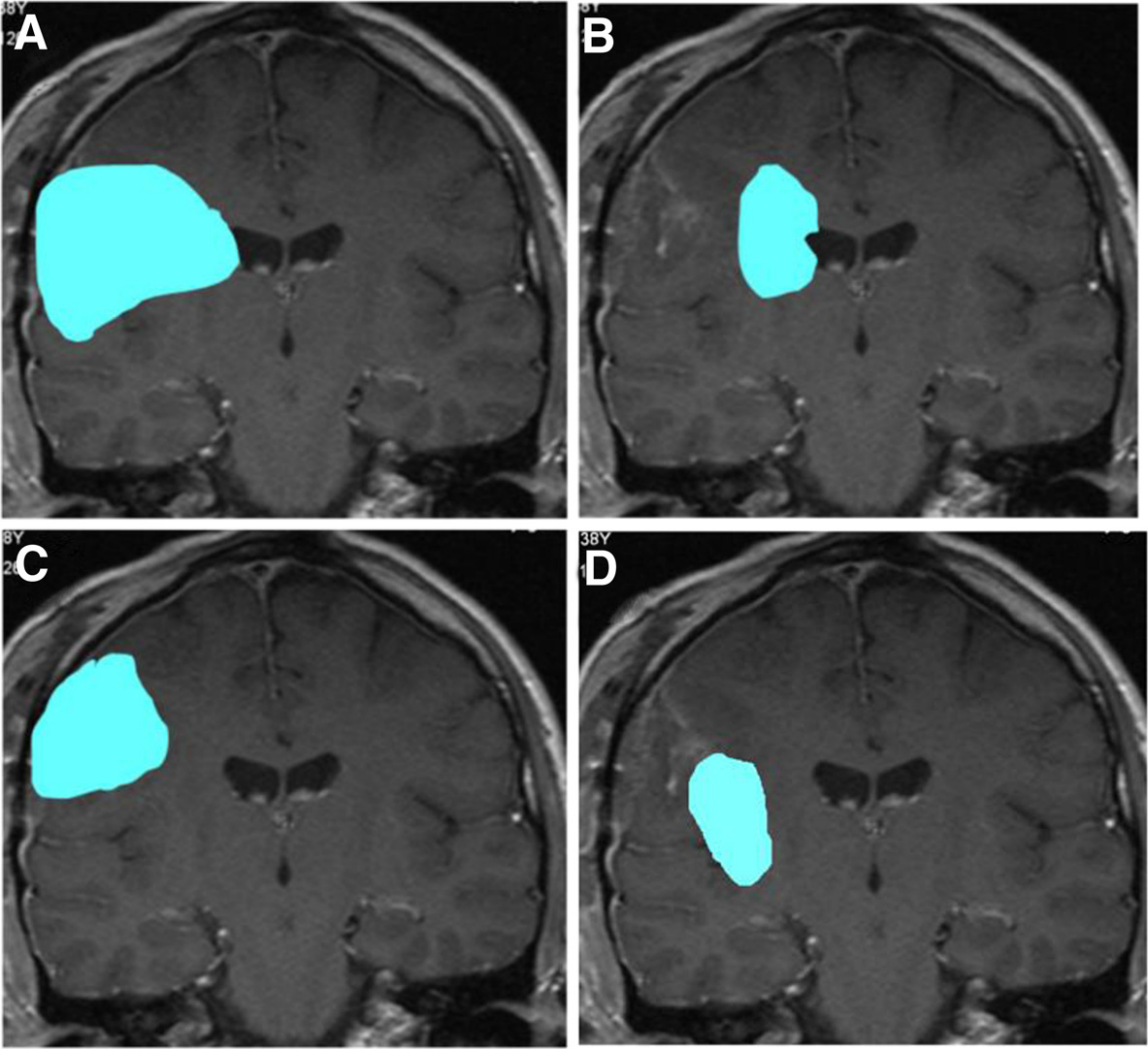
Right temporal planning target volume (PTV, red) and organs at risk delineated in the MRI rendering volume data set. Lenses in pale blue and purple, nn. optici and chiasm in yellow, brain stem and medulla oblongata in green, the hippocampi and the subventricular zones (SVZ) in different shades of magenta.
